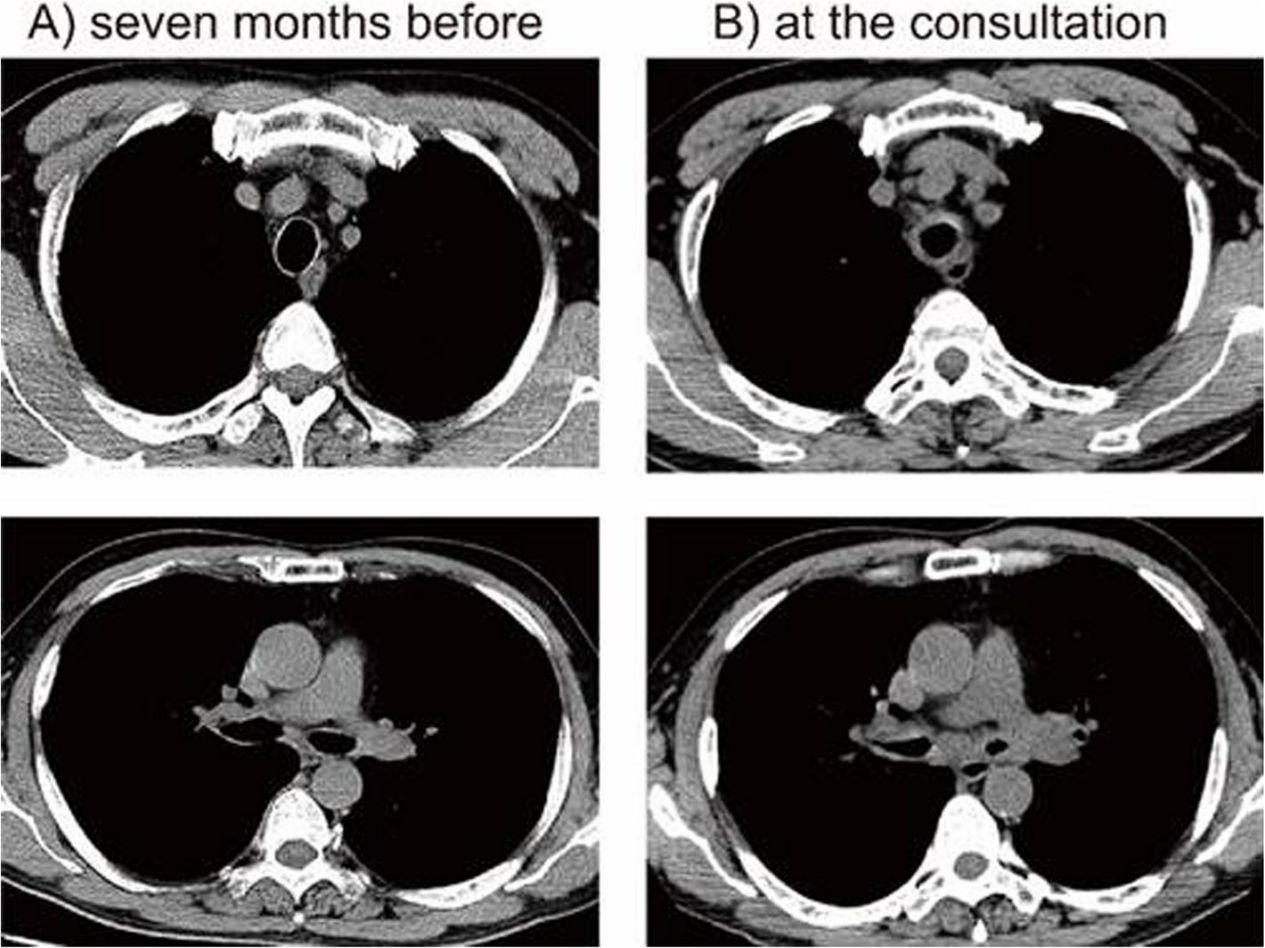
Computer tomography (CT) during the clinical course. Thoracic CT 7 months before (a) and at the time of consultation (b). Compared to before the onset, the wall of the trachea to the bronchiole had thickened diffusely
